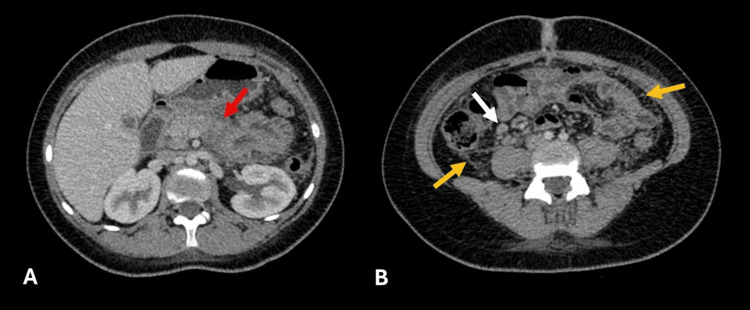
Contrast-enhanced CT (abdomen): Increased pancreatic thickness with heterogeneous enhancement (A, red arrow). Peritoneal and mesenteric micronodules mimicking carcinomatosis (B, yellow arrows), with reactive mesenteric lymph nodes (B, white arrow).
